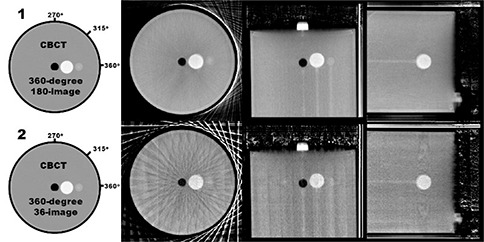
Contrasting sphere slices in the axial, coronal and sagittal planes (from left to right, respectively) of reconstructions based on the CBCT sweep configurations.
